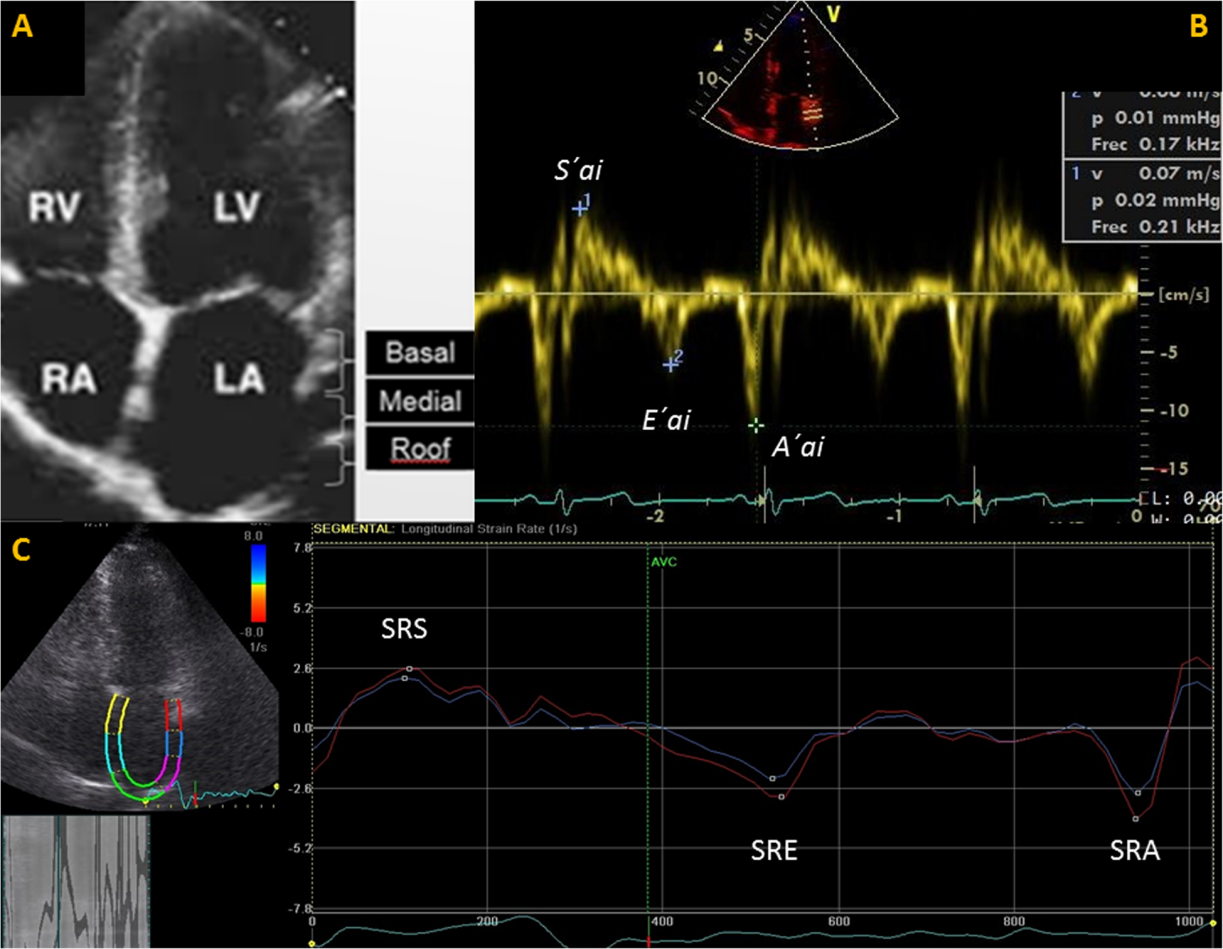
Methodology used for the evaluation of LA lateral wall ​​by TDI and SRI. A) Subjective division of the LA lateral wall in three segments; B) TDI on line by spectral pulse at basal segment of LA lateral wall. Three waves are identified: *S’la* (evaluates reservoir function), *E’la* (evaluates conduit function), *A’la* (evaluates pump function). C) LA SRI by speckle tracking with the analysis of the basal and mid segments. Three waves are identified: SRS (evaluates reservoir function); SRE (evaluates conduit function) and SRA (evaluates pump function). For more information, refer to the text. LA: left atrial, TDI: Tissue Doppler Image, SRI: Strain Rate Image, *la*: left atrial
